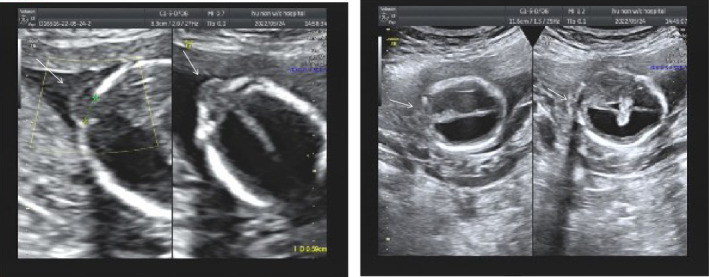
Fetal ultrasound images show the skull defect with an encephalocele (indicated by arrows) at the 17th gestational week in the fourth pregnancy (II:4) of the pregnant mother. NT thickening (6.9 mm) and the enlarged third ventricle were noted.
